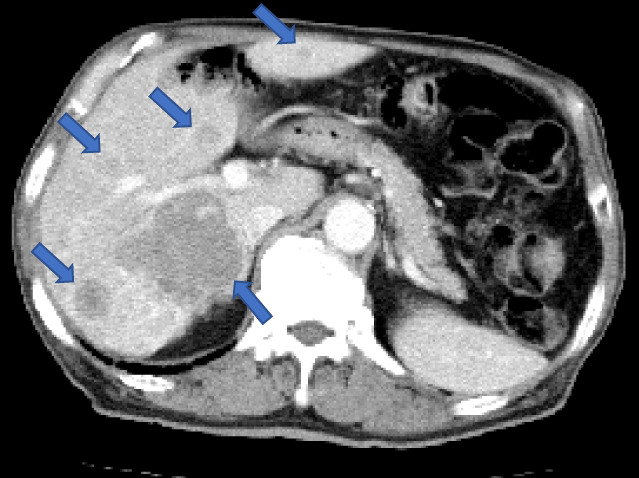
Enhanced computed tomography at diagnosis showing multiple liver metastases. The largest liver metastasis invaded the hepatic capsule with hepatic surface discontinuity.
